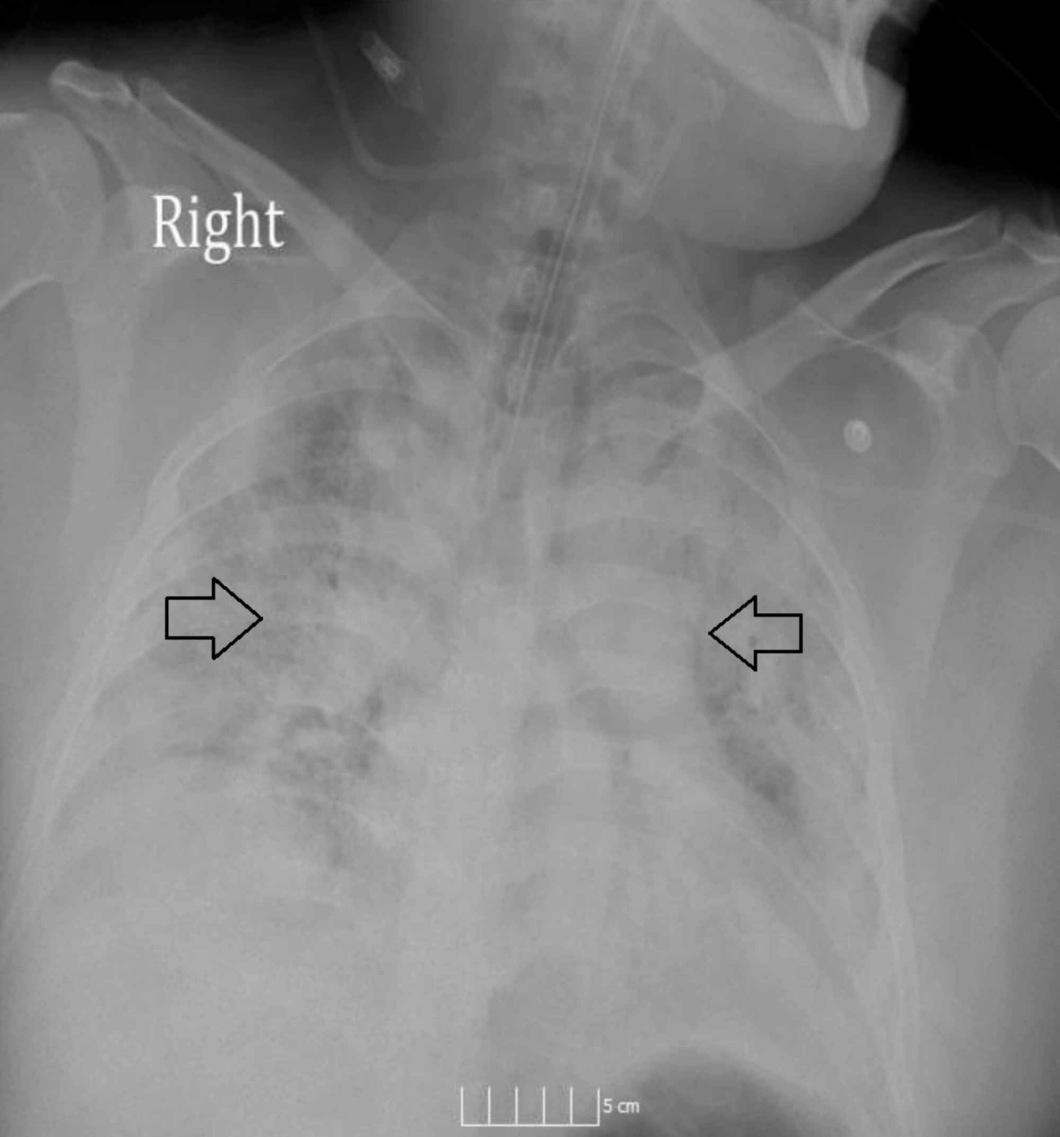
Anteroposterior (AP) chest X-ray showing extensive bilateral airspace disease consistent with acute respiratory distress syndrome (ARDS) on presentation to our hospital.
